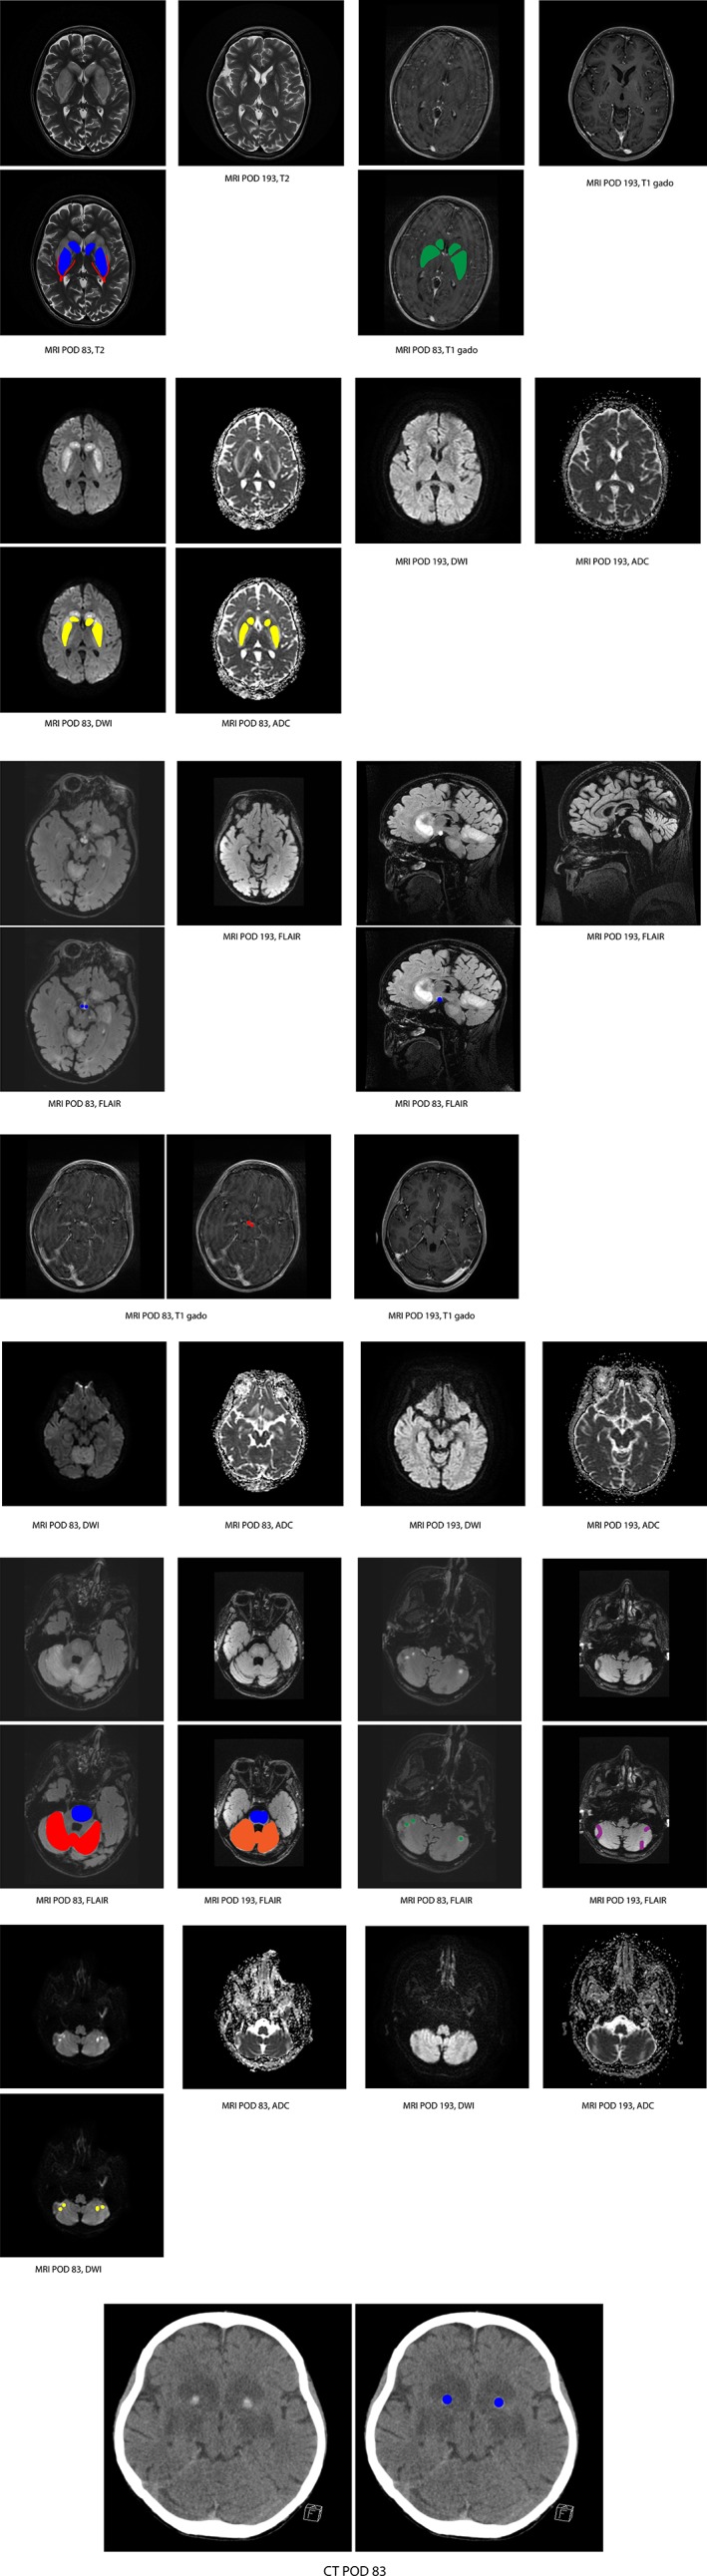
Brain MRI images of case 1 from our center. Post‐operative day (POD) 83 = initial magnetic resonance imaging (MRI) or CT‐scan and POD 193 = follow‐up MRI. FLAIR, fluid attenuated inversion recovery images, acquired after intravenous administration of contrast medium. T1 gado = T1‐weighted image after gadolinium administration; T2 = T2‐weighted images. A, Initial MRI: swelling and T2 hyperintensity of basal ganglia (blue); lentiform fork (red); faint contrast enhancement (green); high intensity on DWI and low/normal ADC in basal ganglia (yellow). Follow‐up MRI: decreased signal abnormalities of basal ganglia, with tissue loss. B, Initial MRI: swelling and T2 hyperintensity of mammillary bodies (blue); avid contrast enhancement (red) and no diffusion restriction. Follow‐up MRI: decreased signal abnormalities, tissue loss. C, Initial MRI: Pons: central T2 hyperintensity (blue). Upper cerebellum, SCA territory: diffuse T2 hyperintensity and swelling (red). PICA and AICA territory: asymmetrical focal T2 hyperintensities (green). Pons and SCA territory: no diffusion restriction (not shown). Focal lesions in PICA and AICA territory: diffusion restriction (yellow). Follow‐up MRI: Pons: central T2 hyperintensity (blue); SCA territory decreased signal abnormalities (orange), diffuse atrophy; PICA and AICA territory: focal tissue loss (purple). D, Calcifications in anterior limb of internal capsule (blue)
